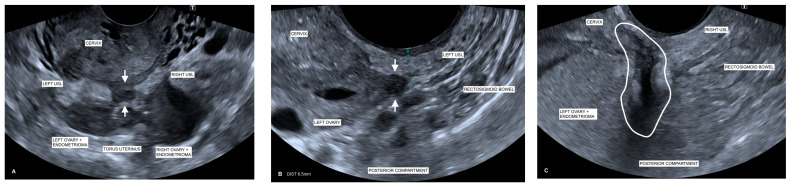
Ultrasound images correlating patient TVS and SST. (A) Endometriomas/medialized ovaries to USLs: Transverse TVS image shows there are bilateral endometriomas and medialization of both ovaries adherent to the USLs (right and left as labeled) at the torus uterinus. A focal endometriotic nodule (arrows) can be seen between the right and left USLs. This patient has confirmed endometriosis associated with long-standing pelvic pain. Correlating SST at this location was described as “sharp” with a total 13/15 pain score. (B) Thickened USL and nodule: USL DE in a woman with severe pelvic pain and dyspareunia who was confirmed to have extensive endometriosis in laparoscopy. Sagittal TVS image shows an irregular and thickened left USL (labeled with caliper measuring 6.5 mm) with adherence of the left ovary (labeled). There is an associated focal endometriotic nodule (arrows) adherent to the adjacent structures. Calipers demonstrate a 6.5 mm thickness of the left USL measured at the thickest point. Correlating SST at this location was described as “sharp” with a total 12/15 pain score. (C) Rectosigmoid DE adherent to USL: Sagittal TVS image demonstrating extensive deep infiltrative endometriosis. Endometriosis plaque deposits (circled) can be seen at the level of the rectosigmoid bowel, tethered to the inferior margin of the left ovary (labeled). The left ovary is medialized, with an endometrioma adherent to the torus uterinus, extending to the right USL. Correlating SST at this location was described as “sharp” with a total 10/15 pain score.
